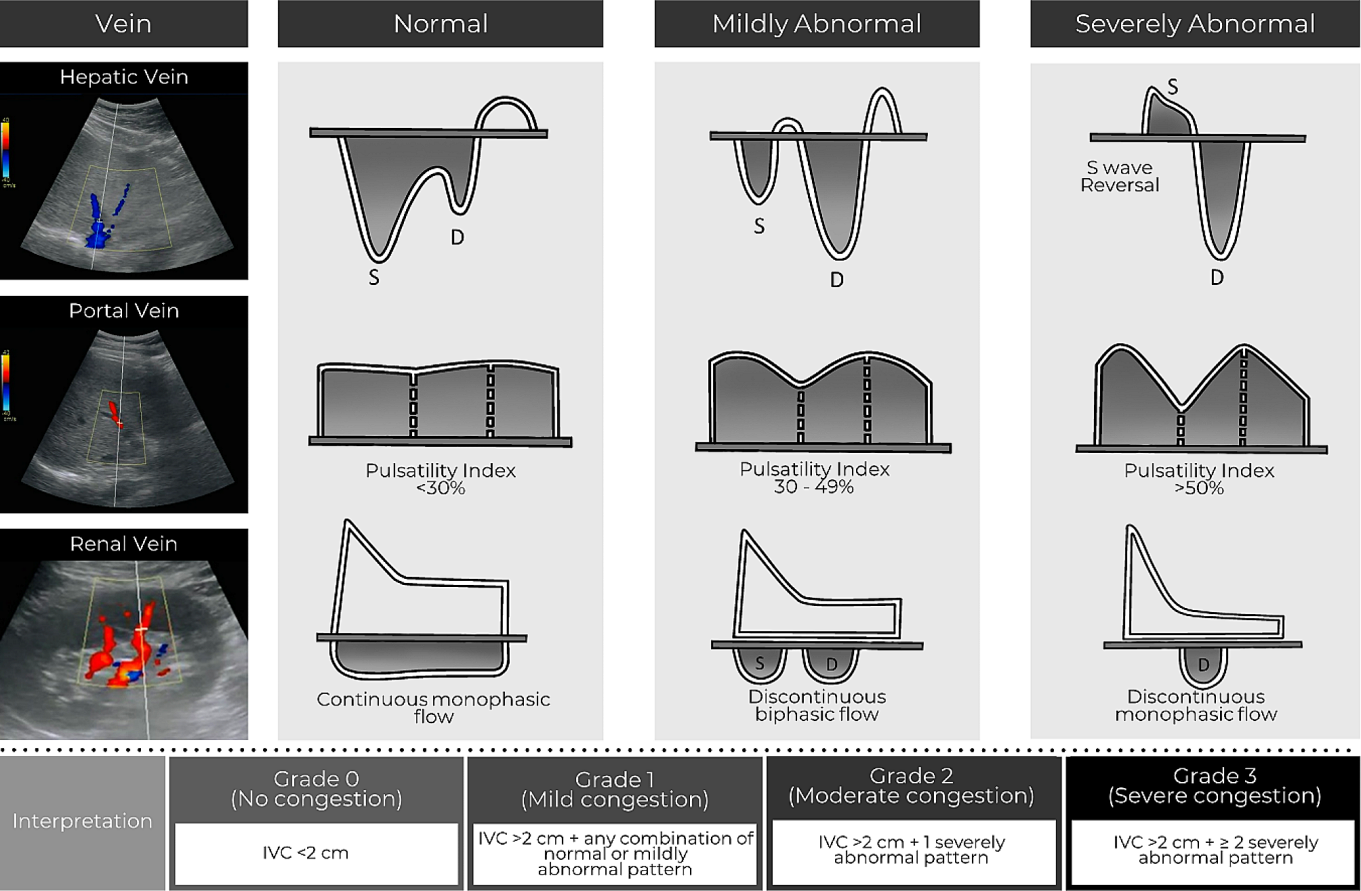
Grading of HV, PV and intrarenal vein Doppler patterns. VEXUS Ultrasound Score: Grade 0: IVC < 2 cm = NO Congestion; Grade 1: IVC > 2 cm with any combo of Normal or Mildly Abnormal Patterns; Grade 2: IVC > 2 cm and ONE severely Abnormal Pattern; Grade 3: IVC > 2 cm and > 2 Severely Abnormal Patterns
